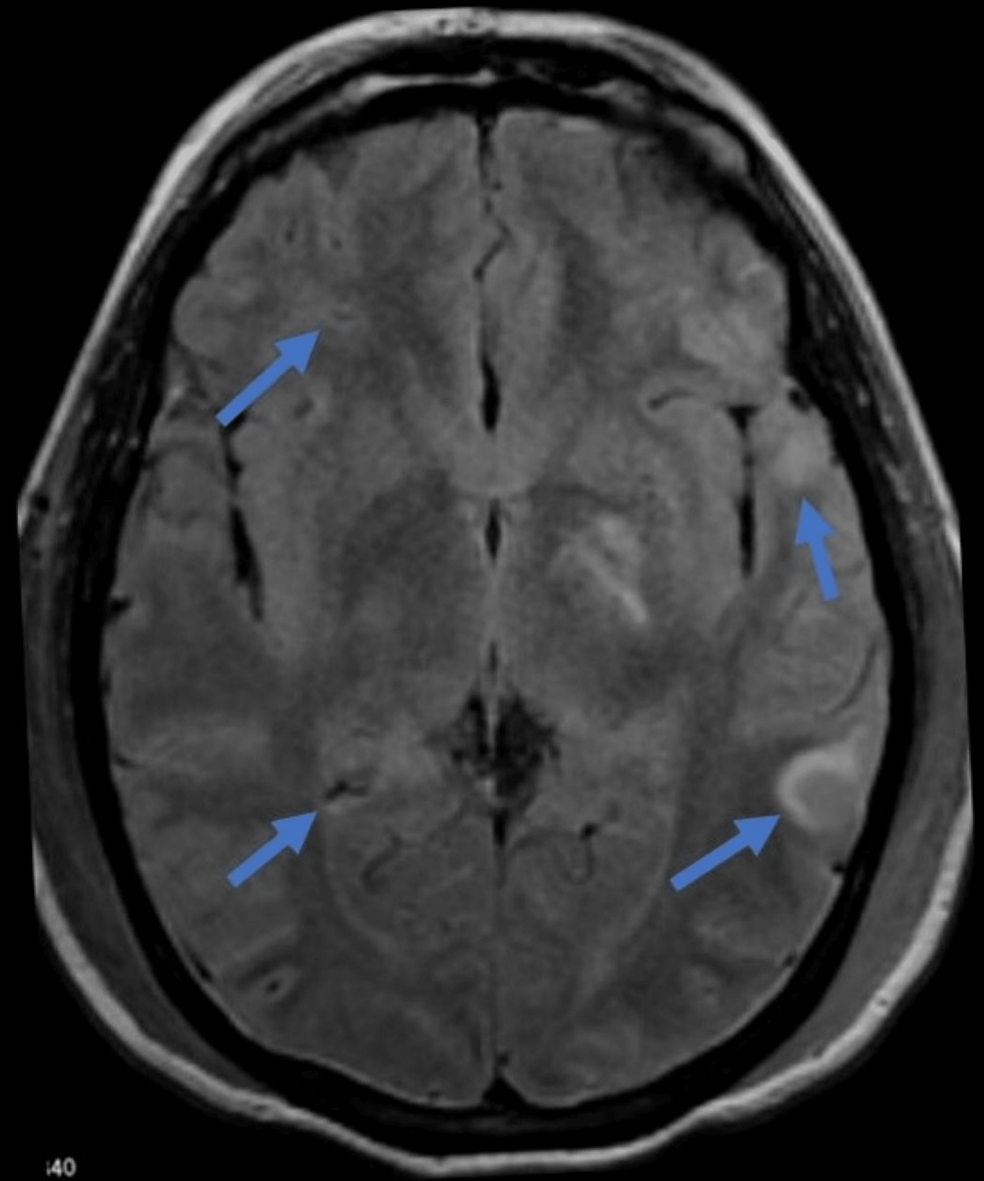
Brain MRI demonstrating diffuse white matter abnormalities and multiple small punctate hemorrhages suggestive of microangiopathy. MRI: magnetic resonance imaging.
